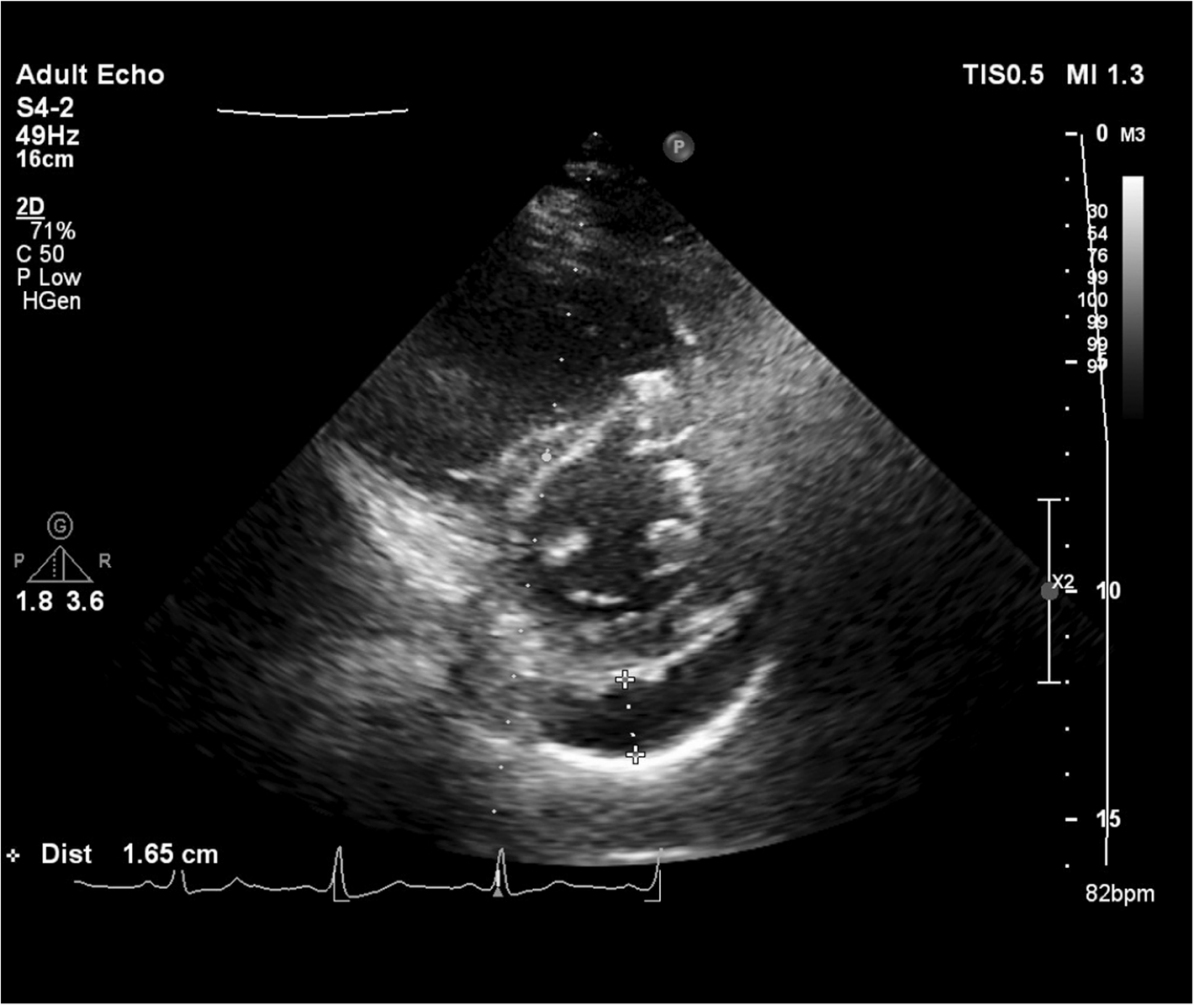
Increased PE in the third patient after ASO implantation was seen in parasternal long axis TTE view
